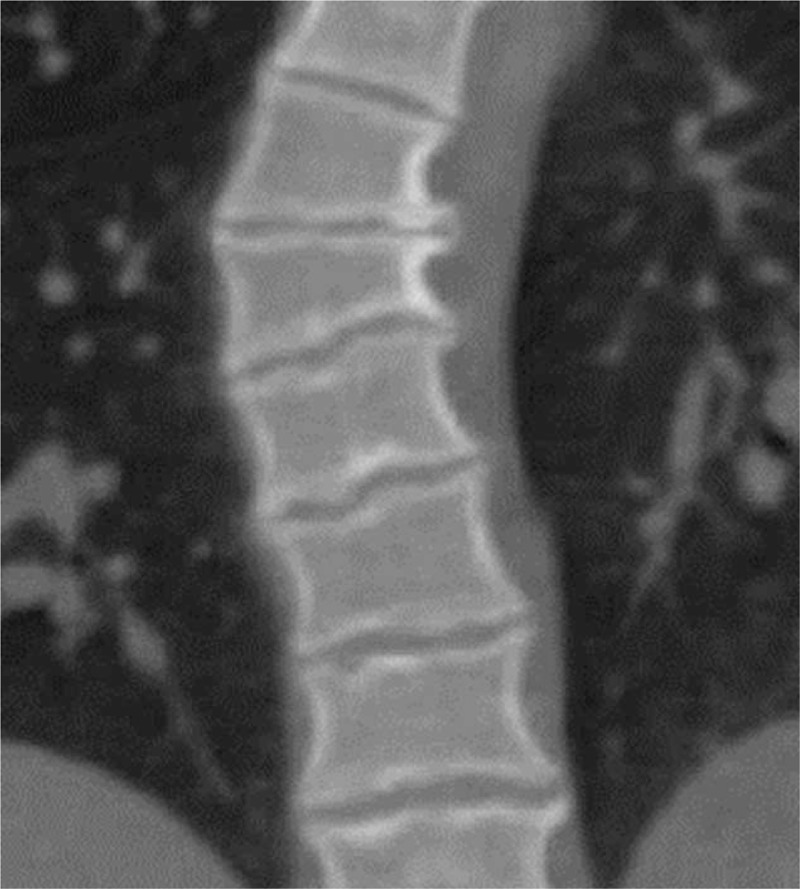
Sagittal 3D-CT scan of the spine of the mother showed trapezoid/wedge-shaped vertebral bodies at the T5–9 associated with irregularities of the vertebral apophyses, sclerosis, Schmorl node (arrow) with subsequent development of osteophytes around the vertebrae causing effectively the development of spinal osteochondritis. CT = computed tomography.
